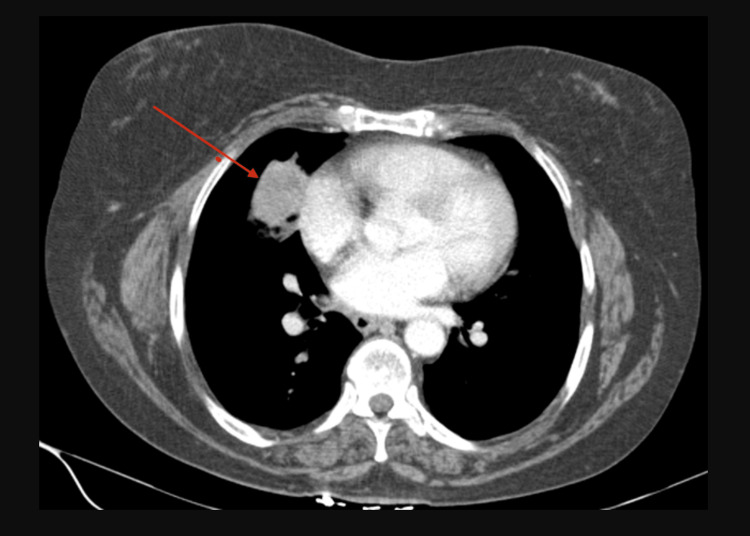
Contrast-enhanced computed tomography of the chest in axial view performed for initial staging showing a heterogeneously enhancing, lobulated, non-calcified right middle lobe mass closely abutting the cardiac border and measuring 4.0 × 3.1 × 2.3 cm. There is mild central low attenuation suggestive of necrosis.
